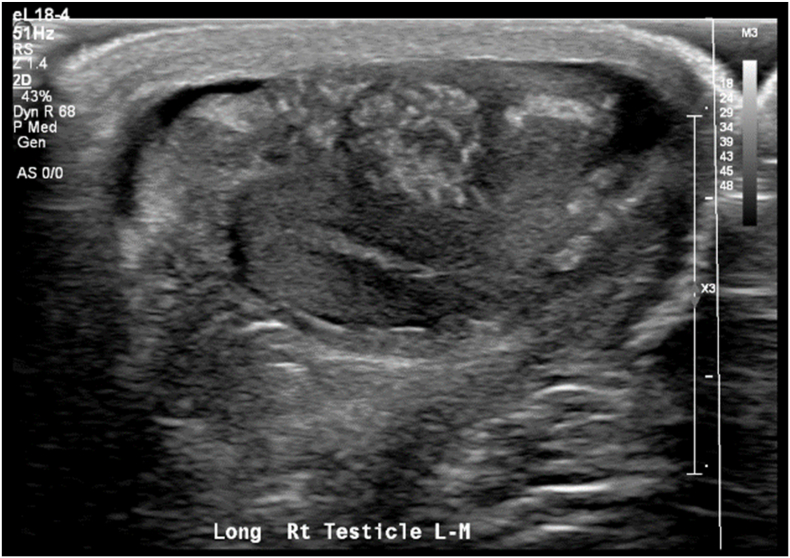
Ultrasound Findings, Epididymal mass with mixed echogenicity and hypervascularity on Doppler.
